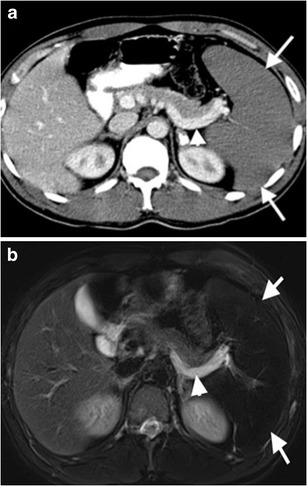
Splenic sequestration. (a) CT features of ASSC. Contrast-enhanced axial CT image of a 26-year-old male with known sickle cell-thalassemia reveals lack of enhancement in spleen parenchyma (*arrow*). Retrograde filling of splenic vein (*arrowhead*) from the portal vein can be visualized. (b) Axial T2-weighted MRI demonstrates hypointense spleen (*arrows*) secondary to iron deposition. Splenic vein manifests with hyperintense appearance (*arrowheads*) due to slow flow resulting from retrograde venous filling
